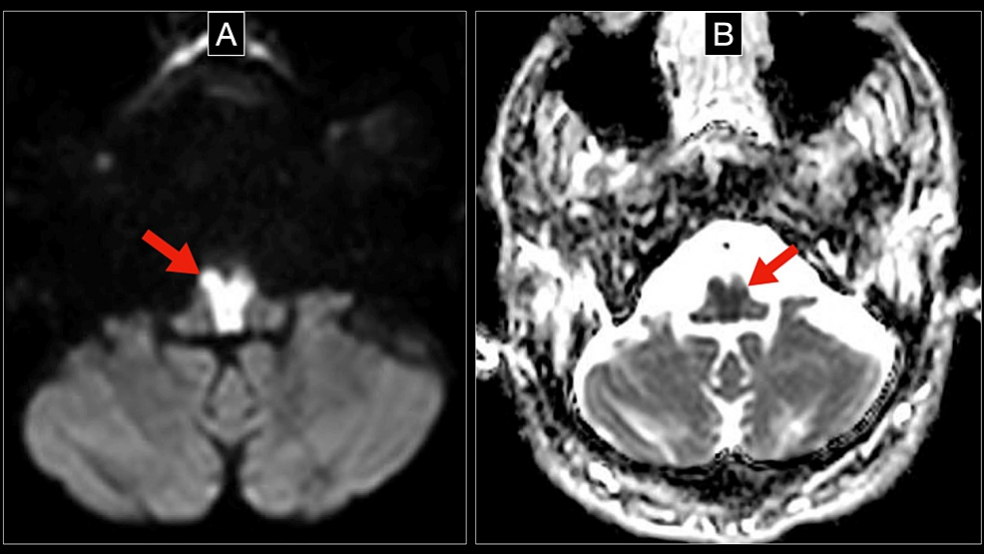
Brain MRI sequences showing an acute bilateral medial medullary infarction Both images are taken from the brain MRI done on the patient a few days after presentation. Figure A (image on the left) shows the DWI sequence at the level of the medulla, and figure B (image on the right) shows the ADC sequence at the same level, both showing the classic "heart sign" (red arrows), characteristic of a bilateral medial medullary infarction. ADC: apparent diffusion coefficient; DWI: diffuse weighted image
